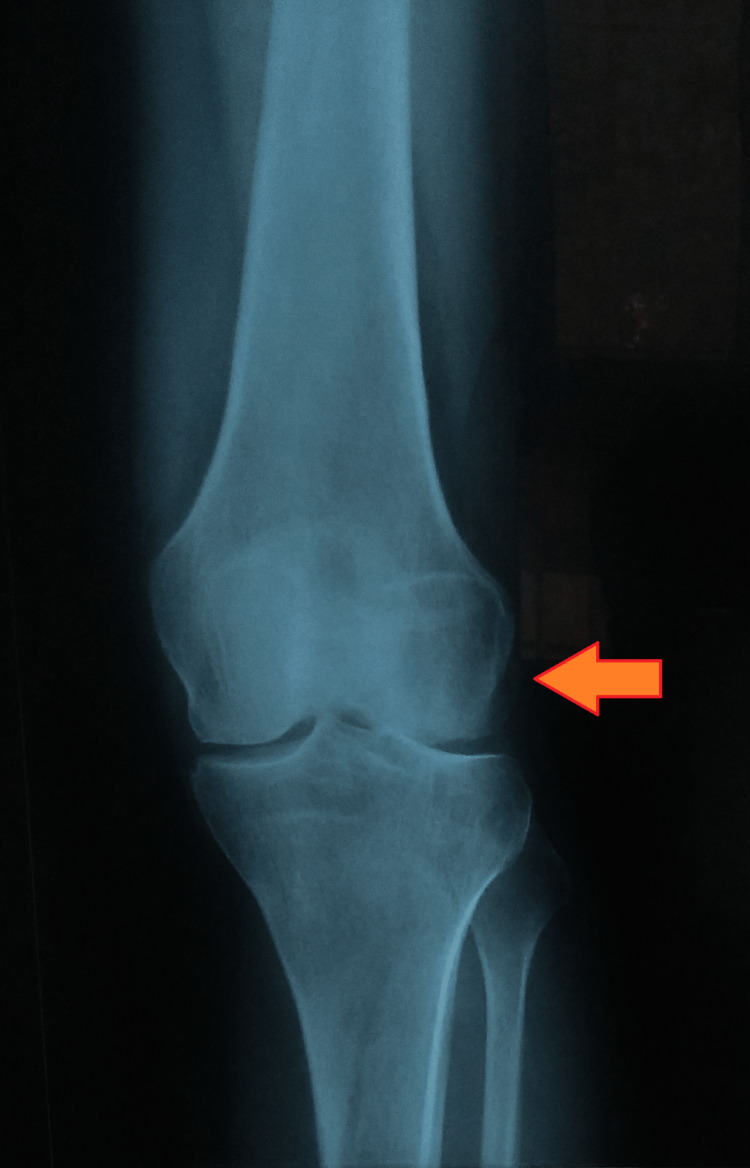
Plain radiograph of the left knee suggestive of bone demineralization of the lateral femoral condyle
